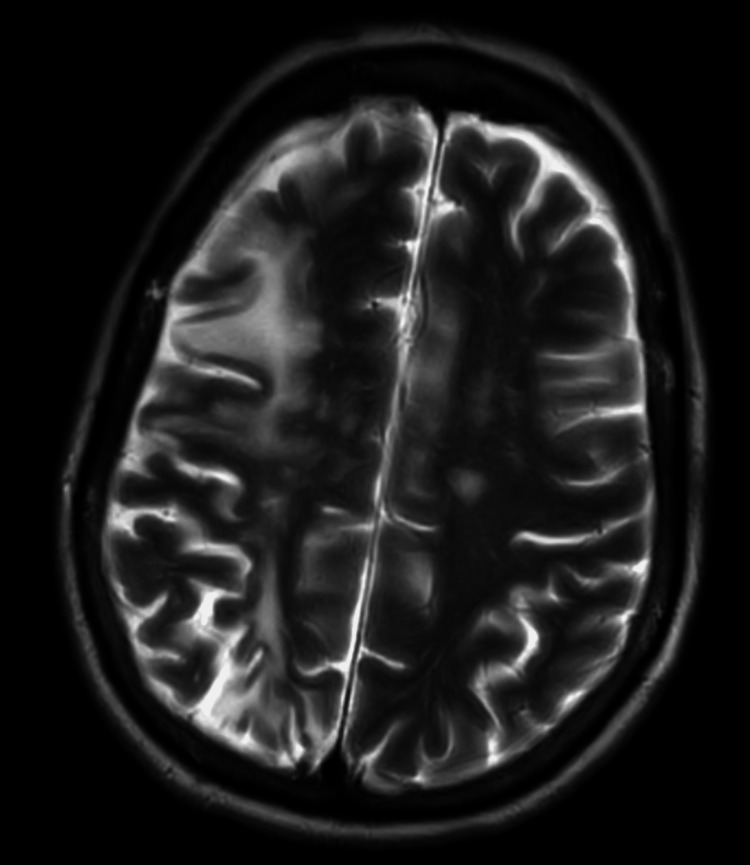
Fluid-attenuated inversion recovery (FLAIR) MRI in the immediate perioperative period. Changes of an old right MCA territory infarction with areas of gliosis and subcortical white matter hyperintensity. Chronic ischemic changes are noted. No evidence of acute intracranial abnormality.
